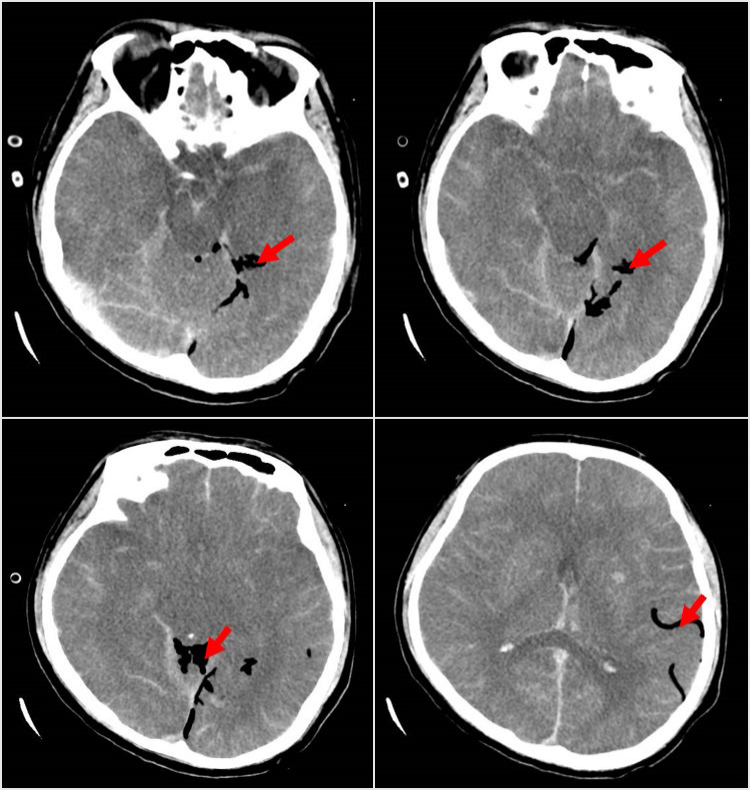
CT scan on day 5 showing exacerbated brain swelling, cerebral herniation, and emerging pneumocrania. Red arrow: visible intracranial pneumatosis. CT: computed tomography.
